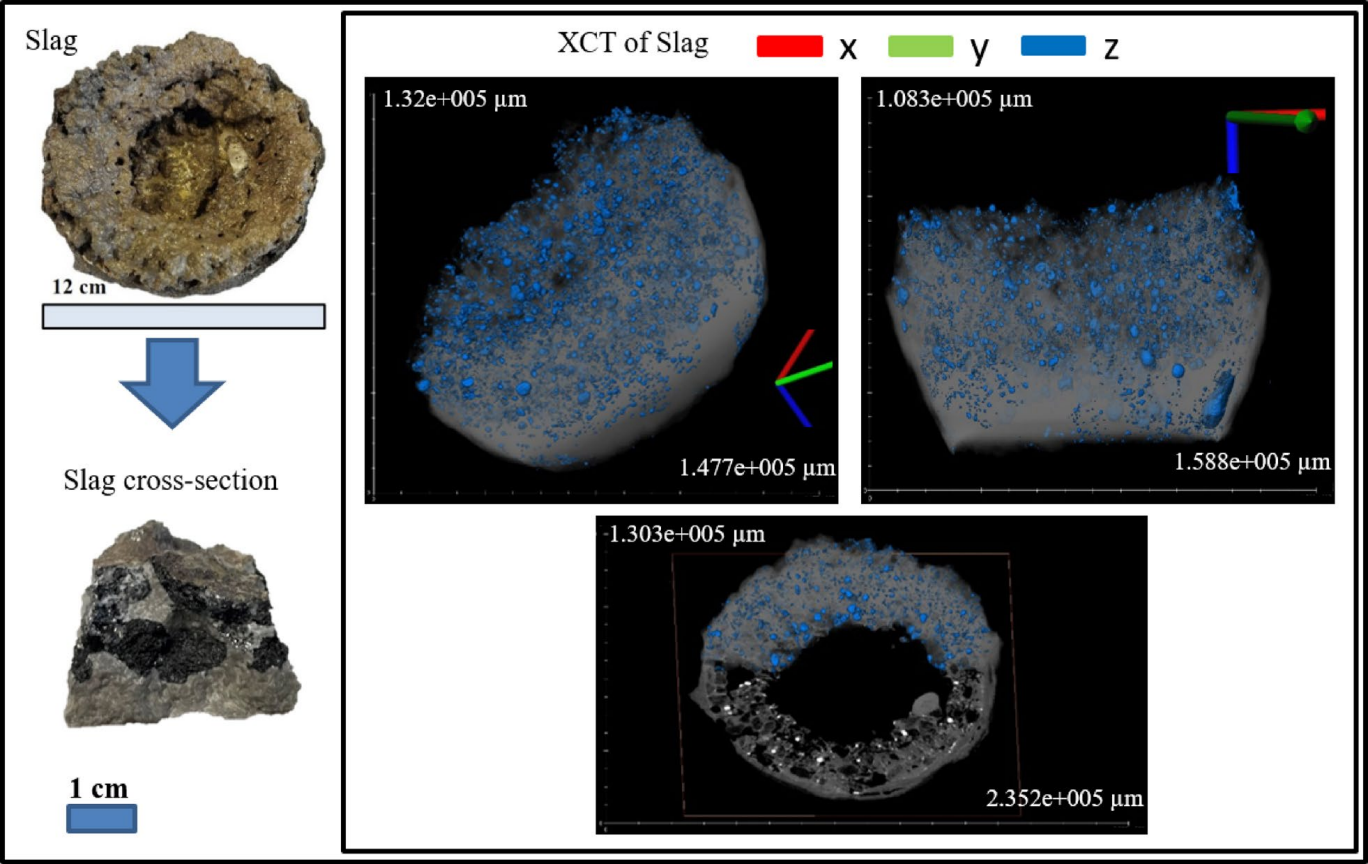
Actual photograph and X-ray computed tomography (XCT) image of slag (gray) and metal droplets (blue).
